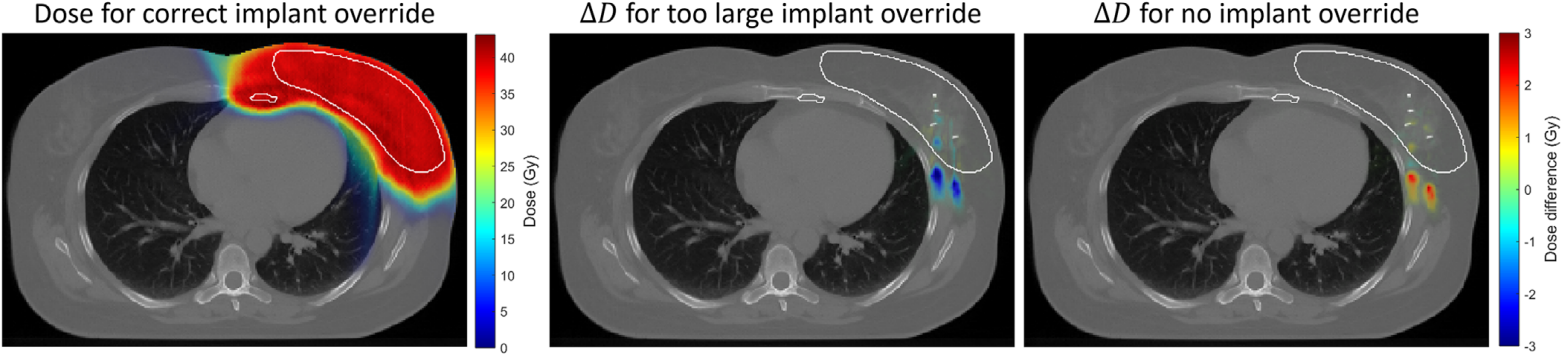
Effect of implant override on the dose distribution—based on the same patient as shown in Figure 1. At the left, the dose distribution on the CT image with the correct implant contour is shown. At the middle and at the right, the dose differences are shown for the CT with a too large contour override (middle) and the CT with no contour override (right). The dose difference is calculated as Δ D = D_toolarge/nooverride_—D_correctovveride_. The white contour shows the CTV. The titanium clips are visible within the contour in the middle and right image (four titanium clips are visible in this CT slice). Dose differences are seen downstream of the clips in both the middle and right images, but with different sign. Also note, the dose differences are generally larger in the middle image for the CT with the too large contour override.
